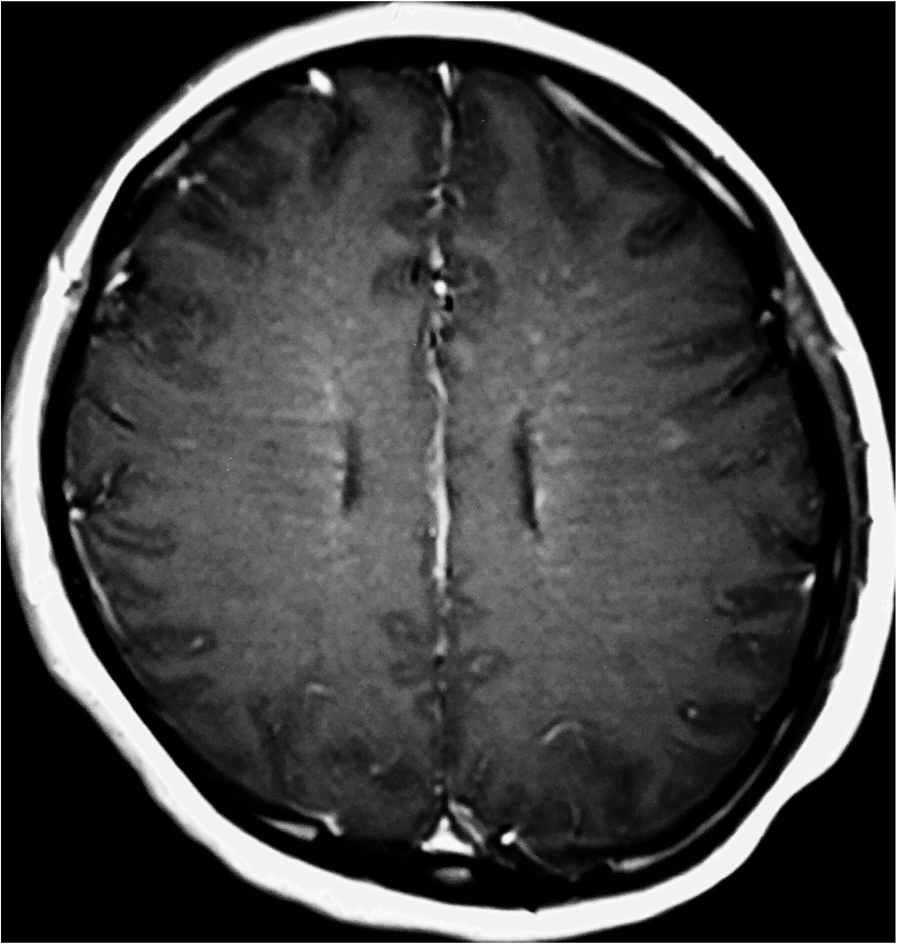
Axial T1 post-contrast sequence MRI showing a radial pattern of linear periventricular post-gadolinium enhancement
